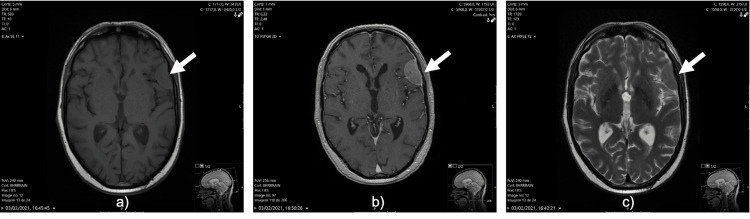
Magnetic resonance imaging showed an extra-axial tumor suggestive of meningioma. (a) Axial T1-weighted images showed a left fronto-opercular, extra-axial lesion with well-defined borders, and isointense to white matter. (b) The lesion showed strong enhancement on post-contrast axial T1-weighted imaging and a dural tail sign. (c) Axial T2-weighted MR images showed that the lesion was slightly hyperintense to white matter; this sequence demonstrated no vasogenic edema in the parenchyma surrounding the lesion.
